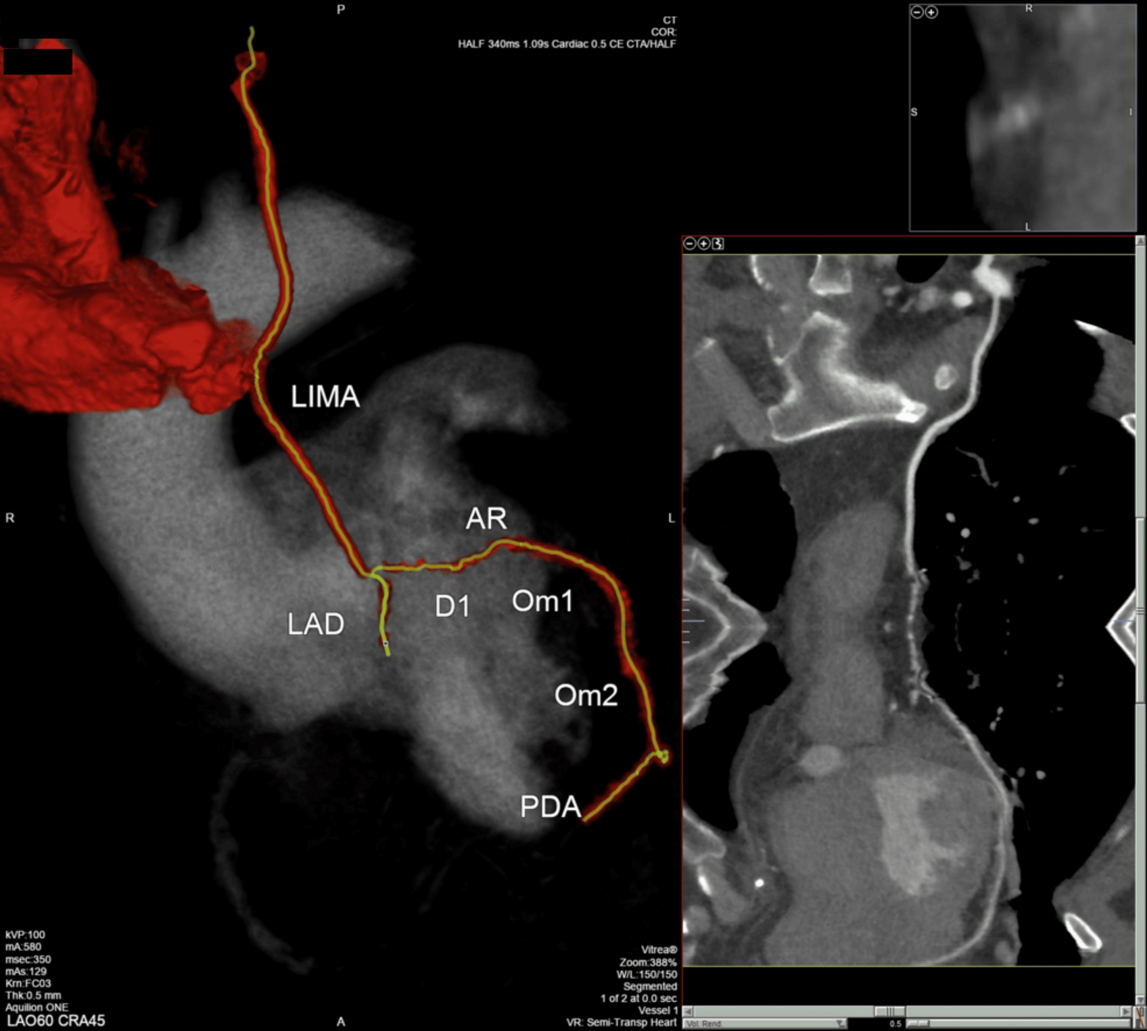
Three months after the operation, a control CT coronarography was performed. In this figure, sequential grafts (LIMA-AR-OM1-OM2-PDA) are visualized. Also, the anastomosis LIMA-DG-LAD is better reflected. LIMA: left intrathoracic mammary artery; AR: radial artery; OM1: first obtuse marginal; OM2: second obtuse marginal; PDA: posterior descending artery; DG: diagonal; LAD: left anterior descending artery
